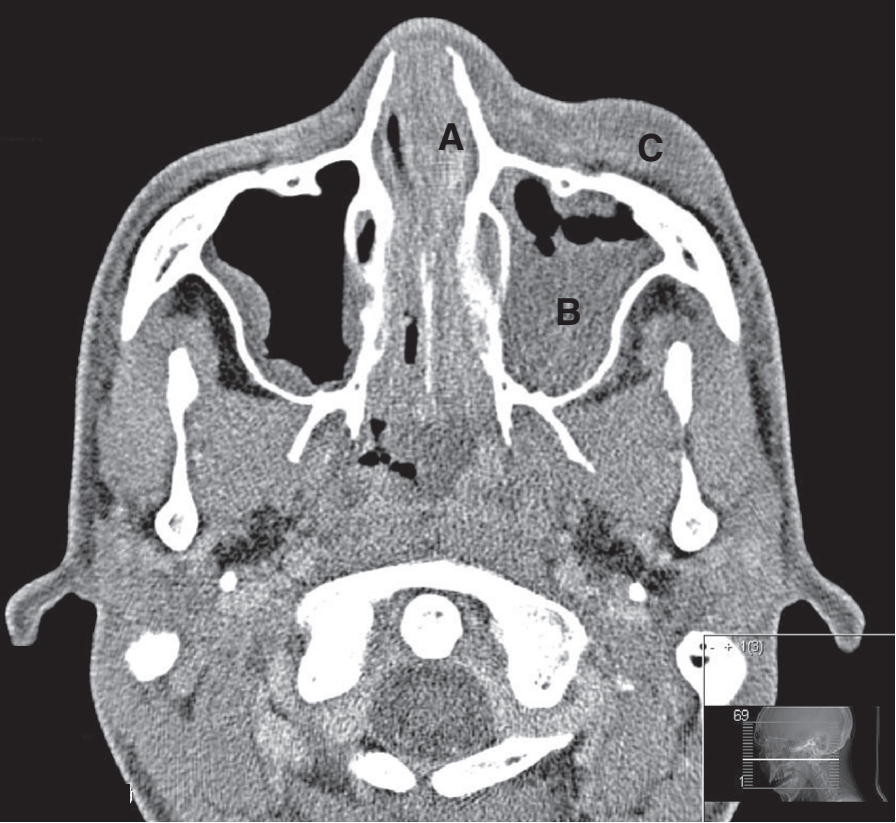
Computed tomography of the head showing A: total opacification of the ethmoid sinus; B: swollen mucosa of the maxillary sinus; C: pre-orbital swelling.
